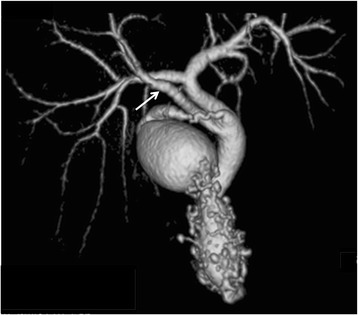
Three-dimensional DIC-CT image (ventral view) showing a type III accessory hepatic duct. The accessory hepatic duct drained the right anterior section (*arrow*) and was classified as belonging to the anterior branch type
